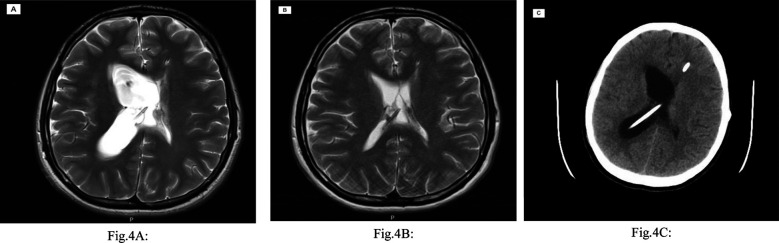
(A & B) MRI brain axial T2 images. (A) A scan obtained before the abdominal US demonstrates dilated entrapped right lateral ventricle due to right-sided VPS malfunction. (B) A scan obtained two days after the abdominal US and demonstrates improved right-sided hydrocephalus. (C) A Plain CT brain scan axial image obtained seven days after abdominal US demonstrates recurrent hydrocephalus related to right-sided VPS malfunction.
